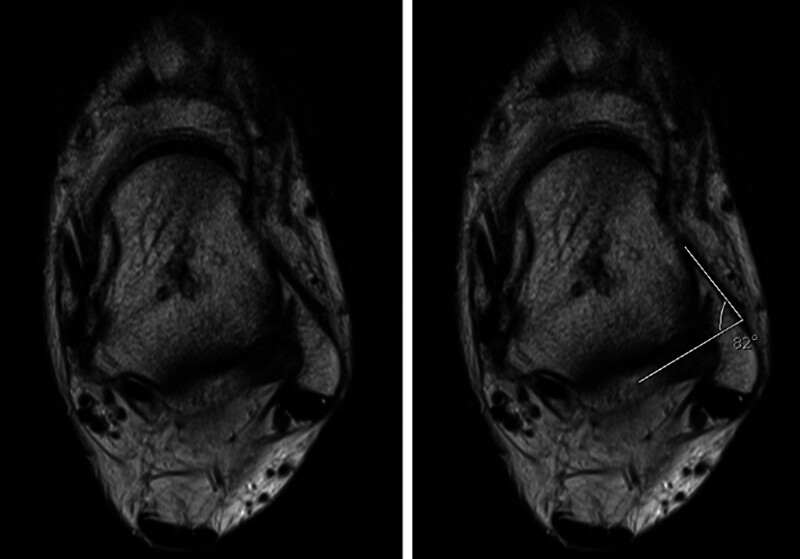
A specific imaging plane was defined from axial T2-weighted images where the anterior talofibular ligament (ATFL) and the posterior talofibular ligament (PTFL) were simultaneously visible. Within this plane, 2 lines were drawn: one parallel to the ATFL and the other parallel to the PTFL. The angle formed by these lines, known as the ATFL–PTFL angle, was measured.
